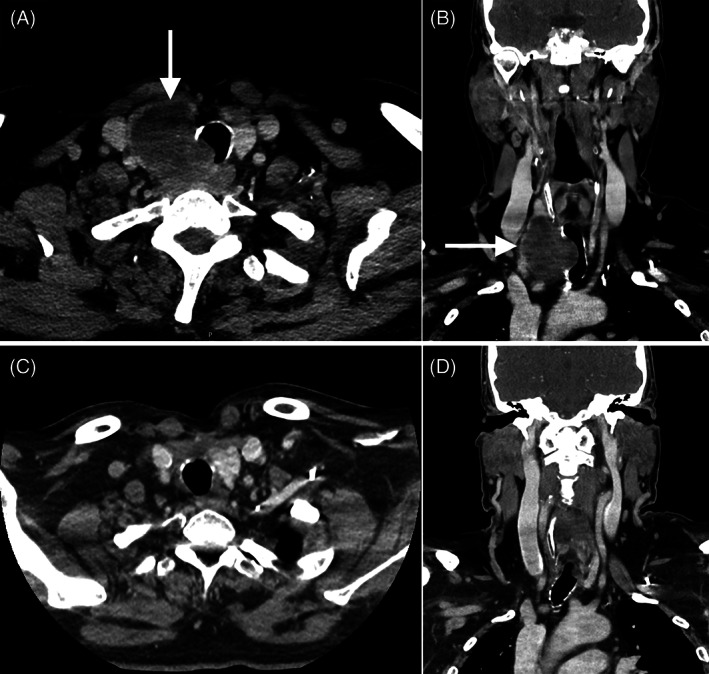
Contrast enhanced axial and coronal computed tomography imaging (A) and (B) on presentation and (C) and (D) at 3 months post‐operative
